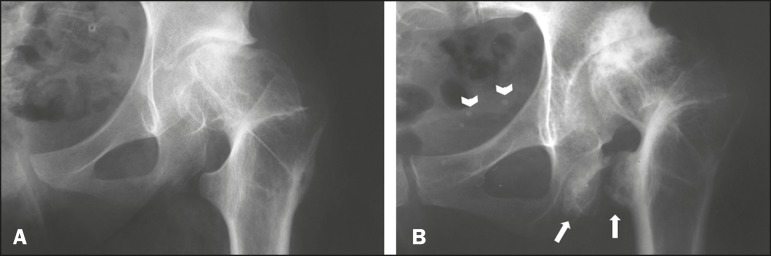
A: Radiograph of the left hip, in an anteroposterior view, approximately
